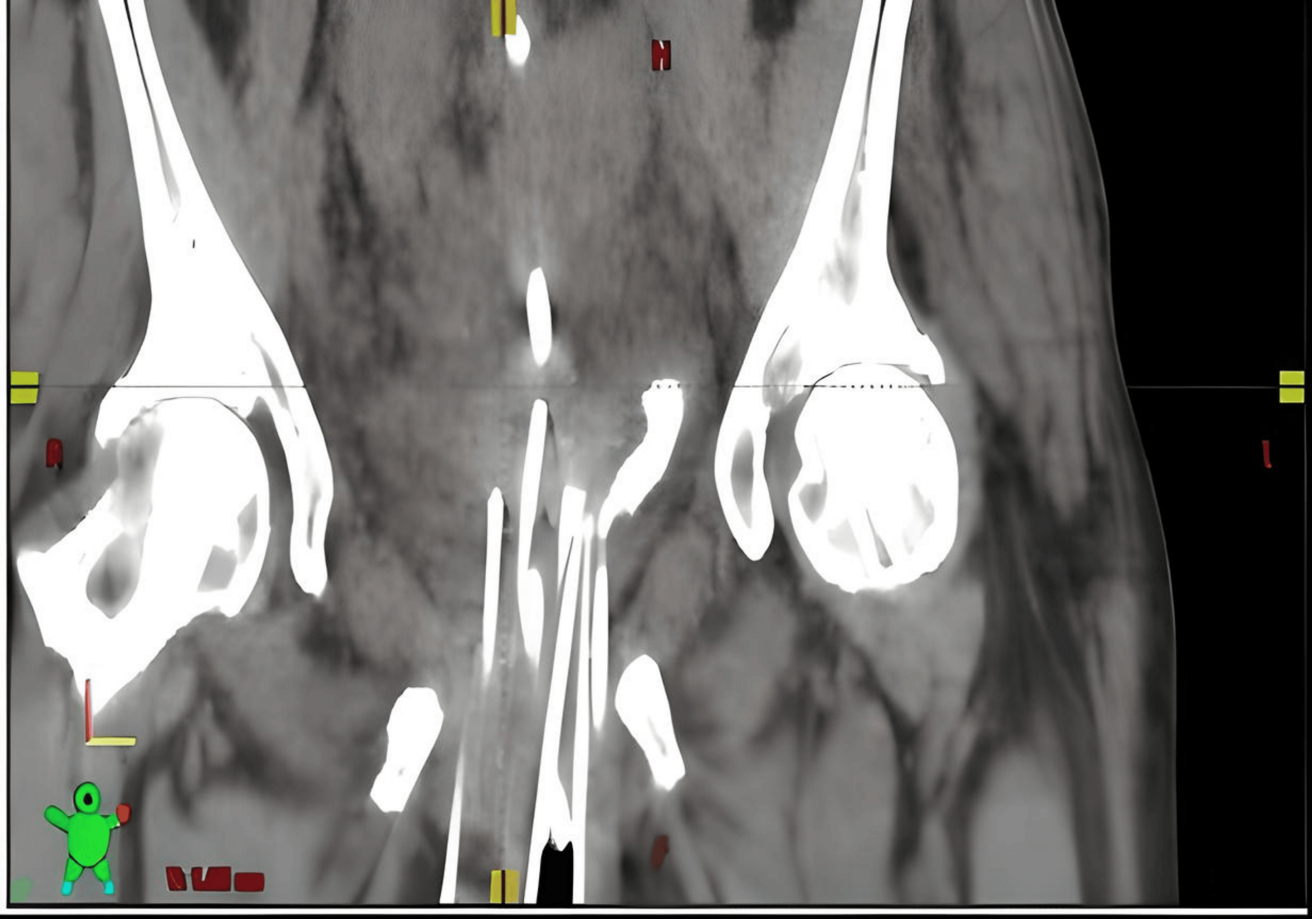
Coronal CT showing Y-tandem
